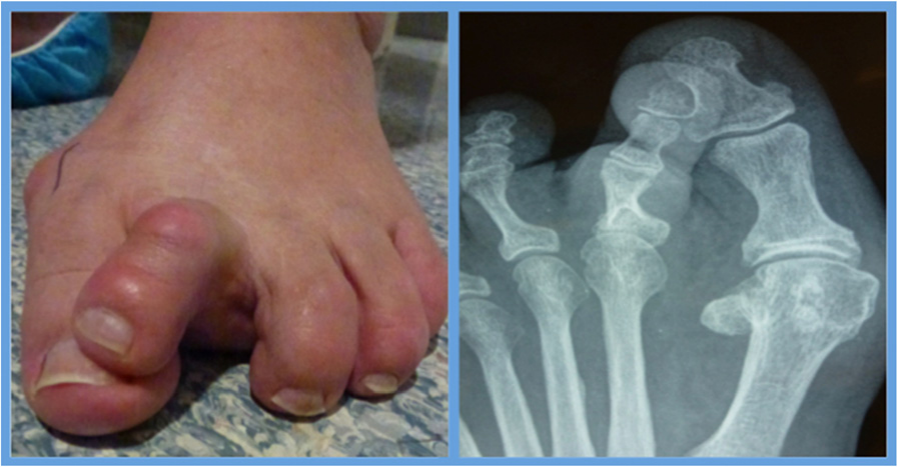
Complex digital deformity (pre-operative). Crossover 2^nd^ toe deformity with subluxation at the MTPJ as seen clinically and radiographically. Note the concomitant hallux valgus deformity.
